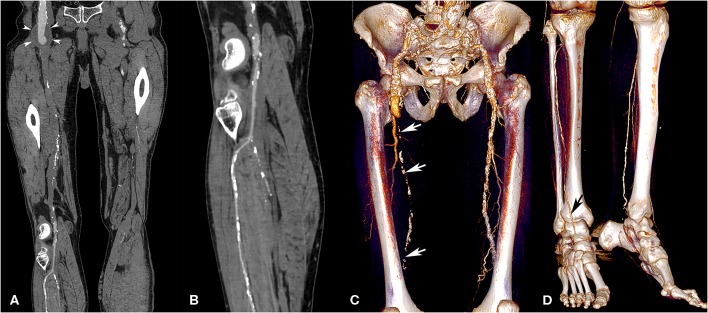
Lower extremity CT angiography obtained with the AA technique in a 75-year-old man with a history of bilateral chronic limb ischemia who had increasing pain on exertion for about a week. Curvilinear reconstructions of the right iliofemoral arterial axis (A) and anterior tibial artery (B) show optimal image quality despite the presence of an aneurysm of the abdominal aorta and right external iliac artery (arrowheads). Volume rendering reconstructions show a long-segment occlusion of the right superficial femoral artery (white arrows, C), yet an optimally enhanced anterior tibial artery (D, black arrow). Attenuation measurements were as follows: aortic level, 235 HU; iliac level, 222 HU (right) and 231 HU (left); femoral level, 188 HU (right), and 233 HU (left); tibial level 235 HU (right), and 218 HU (left); pedal level, 168 HU (right), and 130 (left). HU, Hounsfield units.
